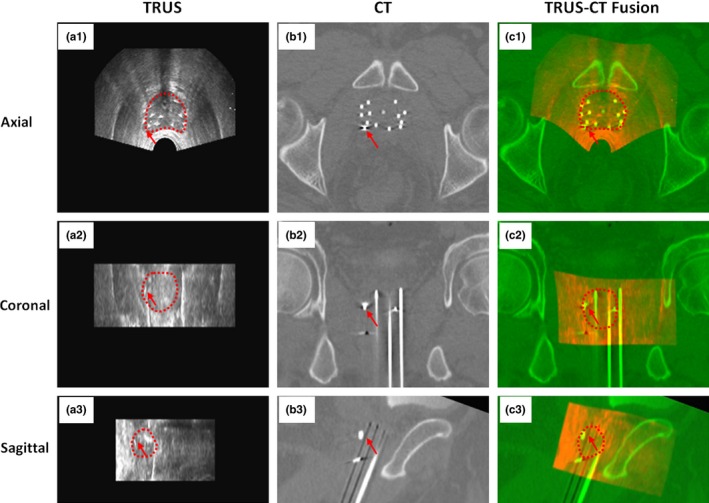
Example of TRUS‐CT registration. A gold marker (arrow) and catheters match well on the TRUS‐CT fusion image.
